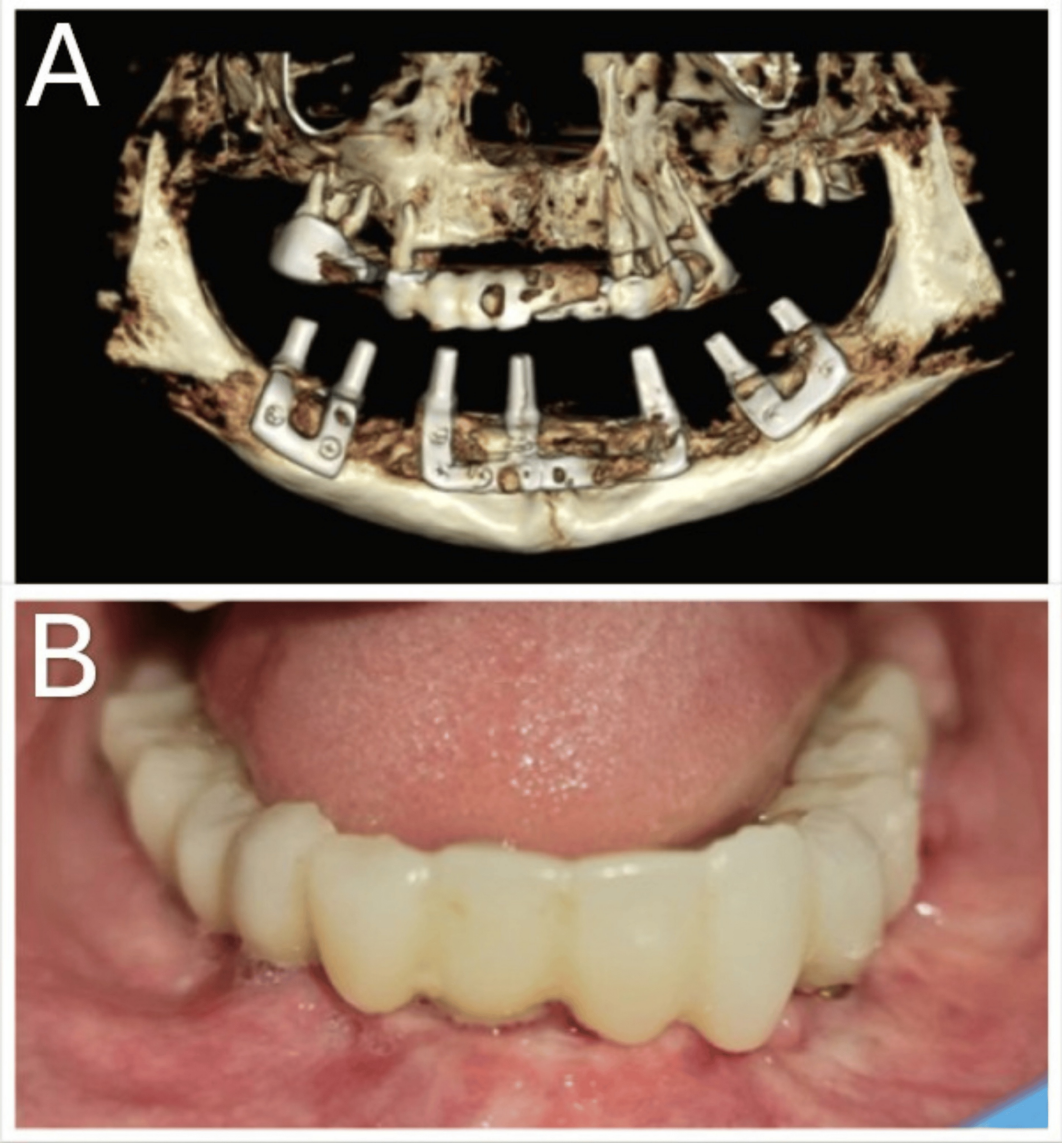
(A) Postoperative CBCT scan and (B) definitive cement-retained, resin-reinforced bridge after six months.
